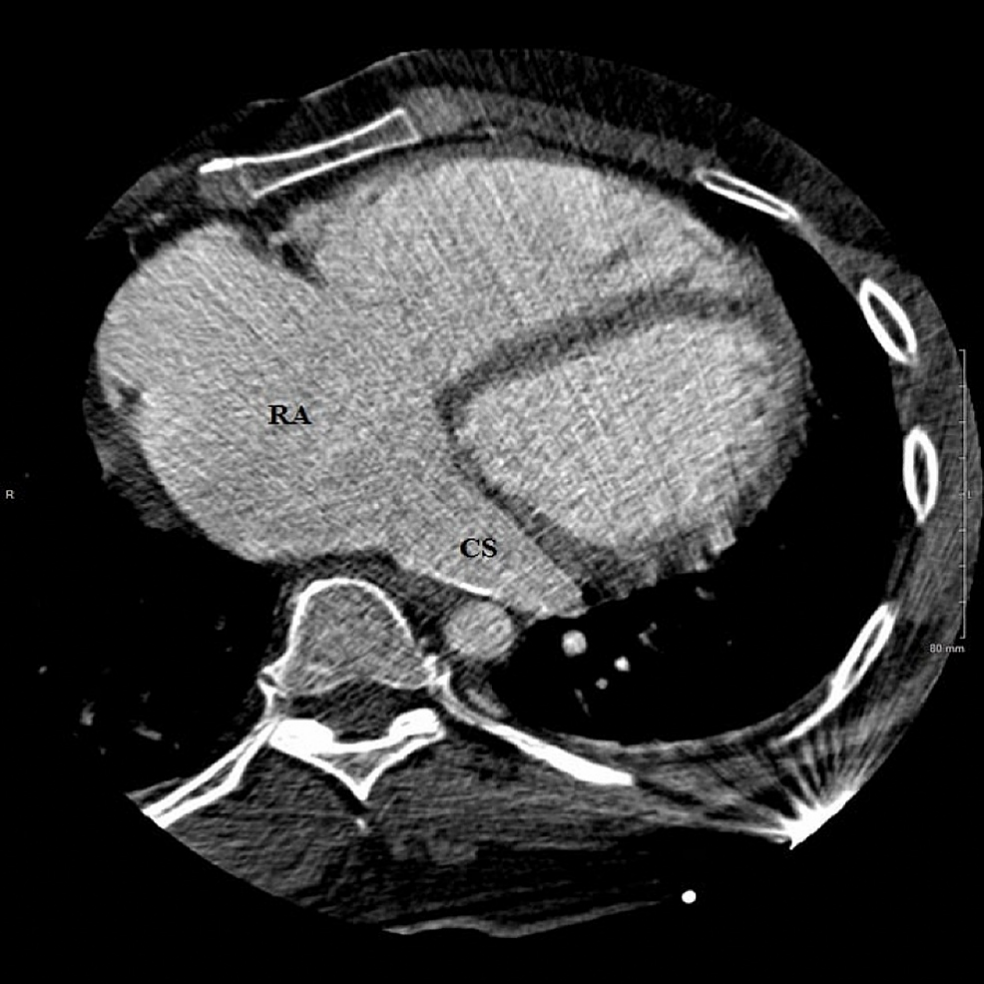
A CT image in the axial plane demonstrates multi-chamber cardiac enlargement. The PLSVC (not imaged) connects to a dilated coronary sinus which drains into the right atrium. PLSVC: persistent left superior vena cava, CS: coronary sinus, RA: right atrium.
